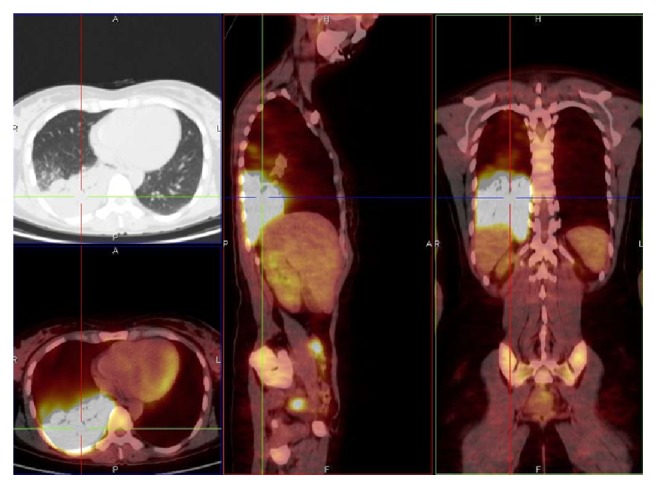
M. tuberculosis* infection. Massive area of high metabolic activity, SUV max 19.55, lower right lobe. Related to patient 11 (PET/CT. Courtesy “V. Monaldi” Hospital).
